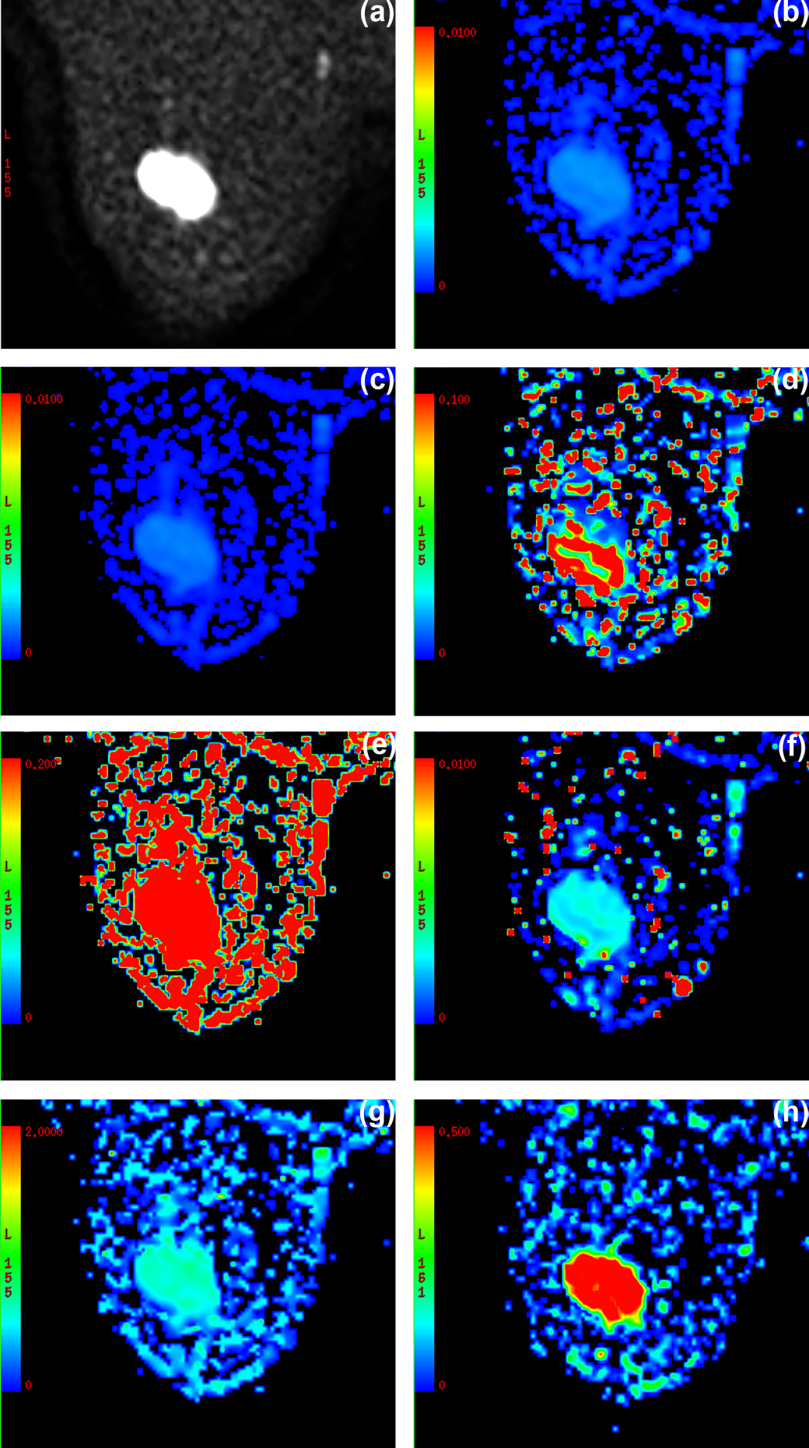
A 48-year-old patient who was pathologically confirmed with benign breast fibroadenomas exhibited a mass on the left breast in the correlating area, measured 10 mm $$\times$$ 13 mm. Its region of interest (ROI) was based on (a) axial STIR-DWI at b-value = 1000 s/mm^2^ with hyperintensity. Parametric maps of (b) average standard ADC: 1.39 × 10^−3^mm^2^/s; (c) ADC_slow_: 1.10 × 10^−3^mm^2^/s; (d) ADC_fast_: 6.14 × 10^−3^mm^2^/s; (e) f: 0.326; (f) DDC: 2.56 × 10^−3^mm^2^/s; (g) α: 0.596 and (h) ADC_AQP_: 0.604um^2^/ms were demonstrated, respectively
